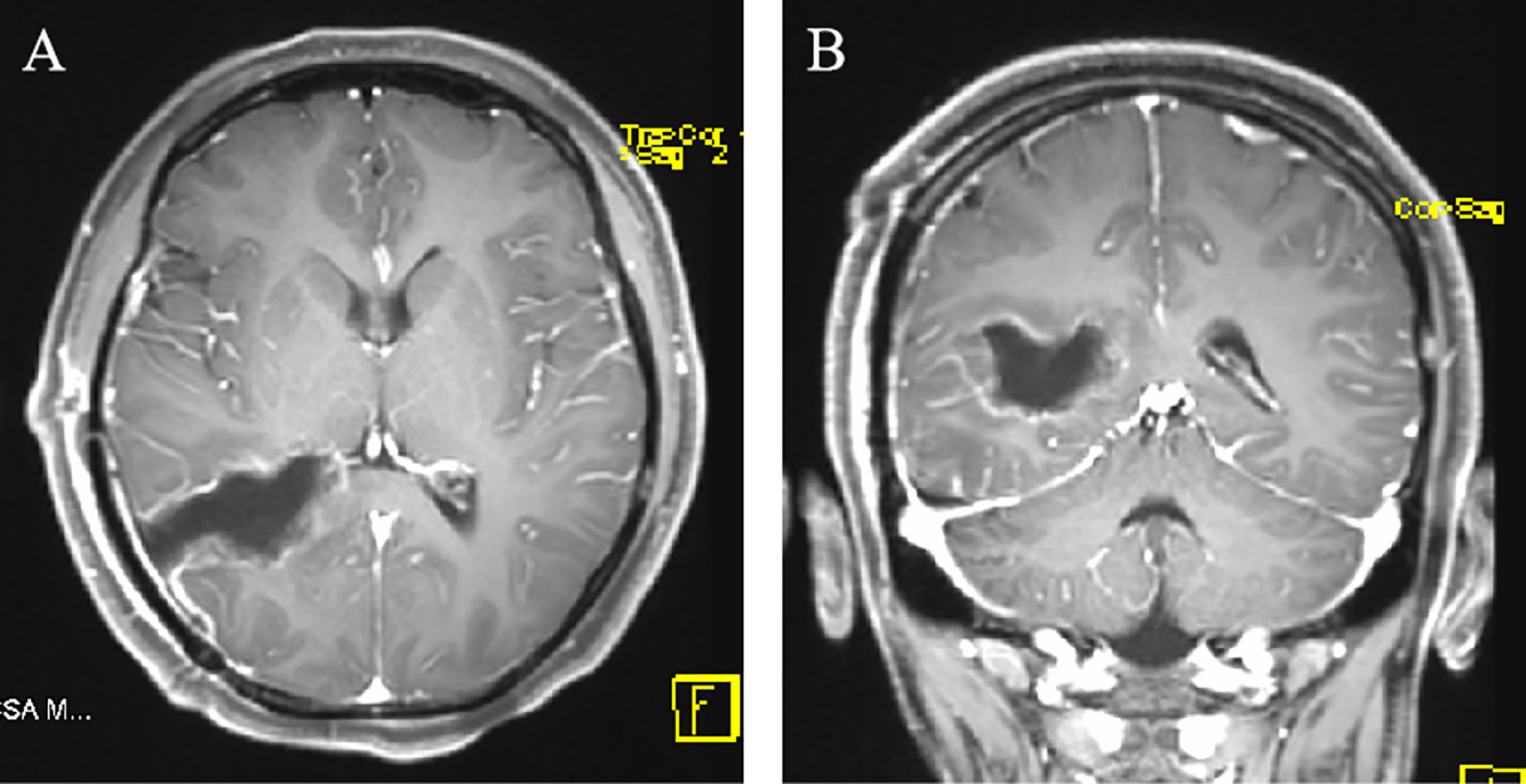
(A, B) Postoperative axial and coronal MRI showing that no parts of the right lateral ventricle lesion remained after resection by a temporal-occipital craniotomy.
